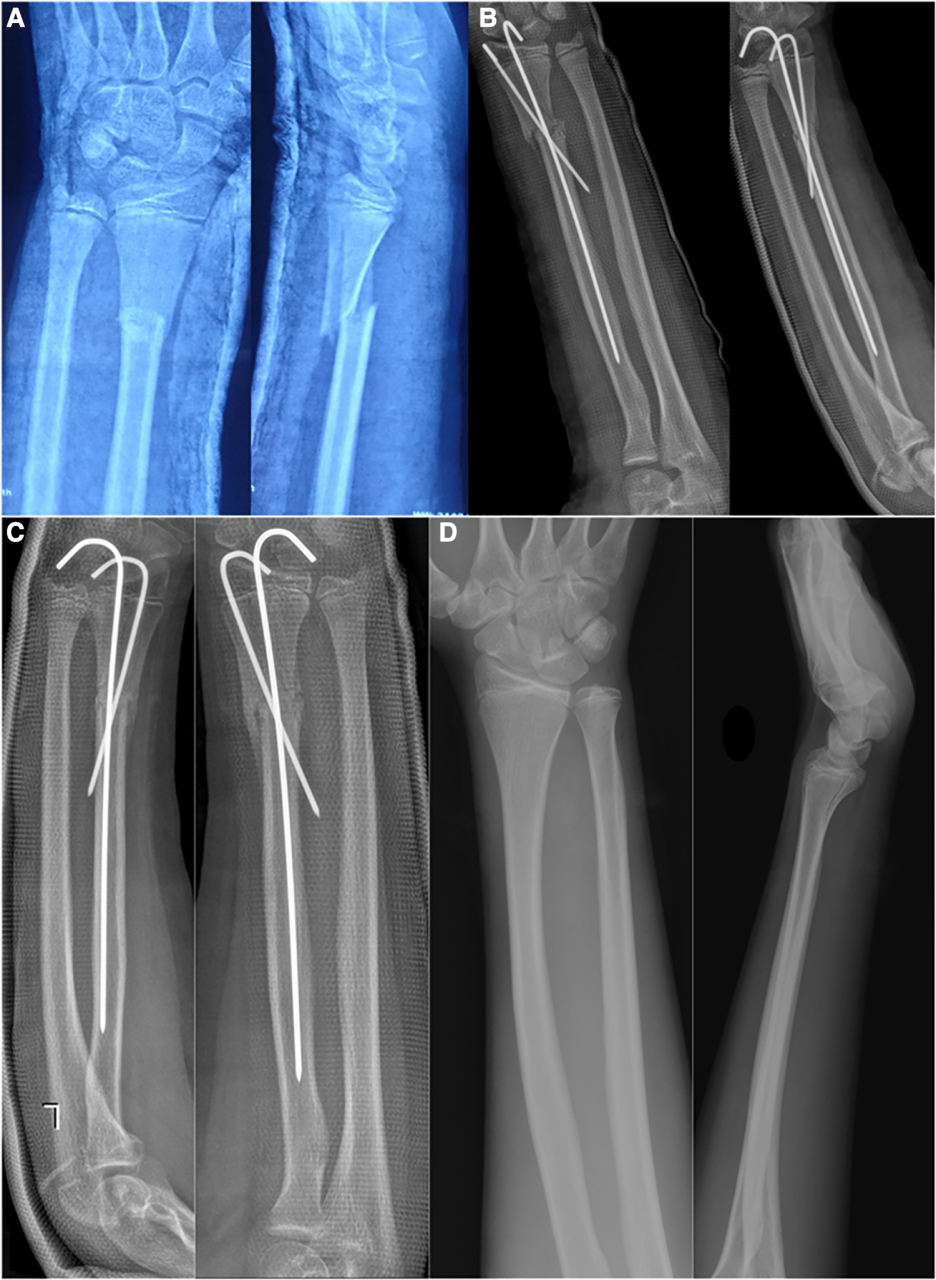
An 11-year-old boy with left DRMDJ fracture. (A) Obvious displacement of DRMDJ fracture on AP and lateral x-ray films. (B). The x-ray film was rechecked immediately after the operation. There was a residual lateral displacement and dorsal angulation. (C) At 6 weeks post-operative, much of the callus formation and the K-wires were removed. (D) Three years after the operation the fracture was completely healed on radiography.
